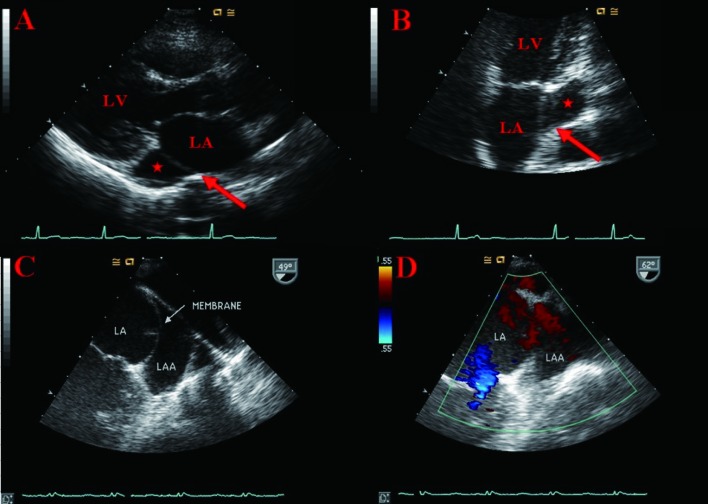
TTE showed left atrium with a linear, mobile, membrane-like structure (arrow) across left atrial appendage (asterisk) (A, B). TEE demonstrated a linear, mobile, membrane-like structure traversing the body of left atrial appendage without a turbulent Doppler color flow across the membrane with Color Doppler (C, D). (LA, left atrium; LV, left ventricle; LAA, left atrial appendage).
